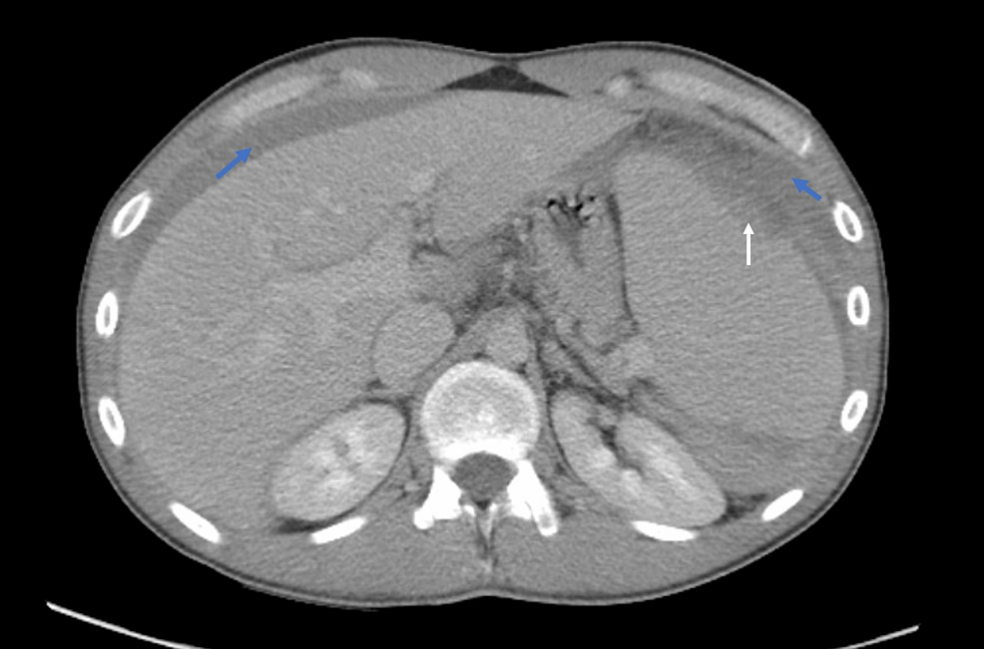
CT scan of the abdomen showing the area of splenic laceration (white arrow) and hemoperitoneum around the spleen and the liver (blue arrow). CT, computed tomography
